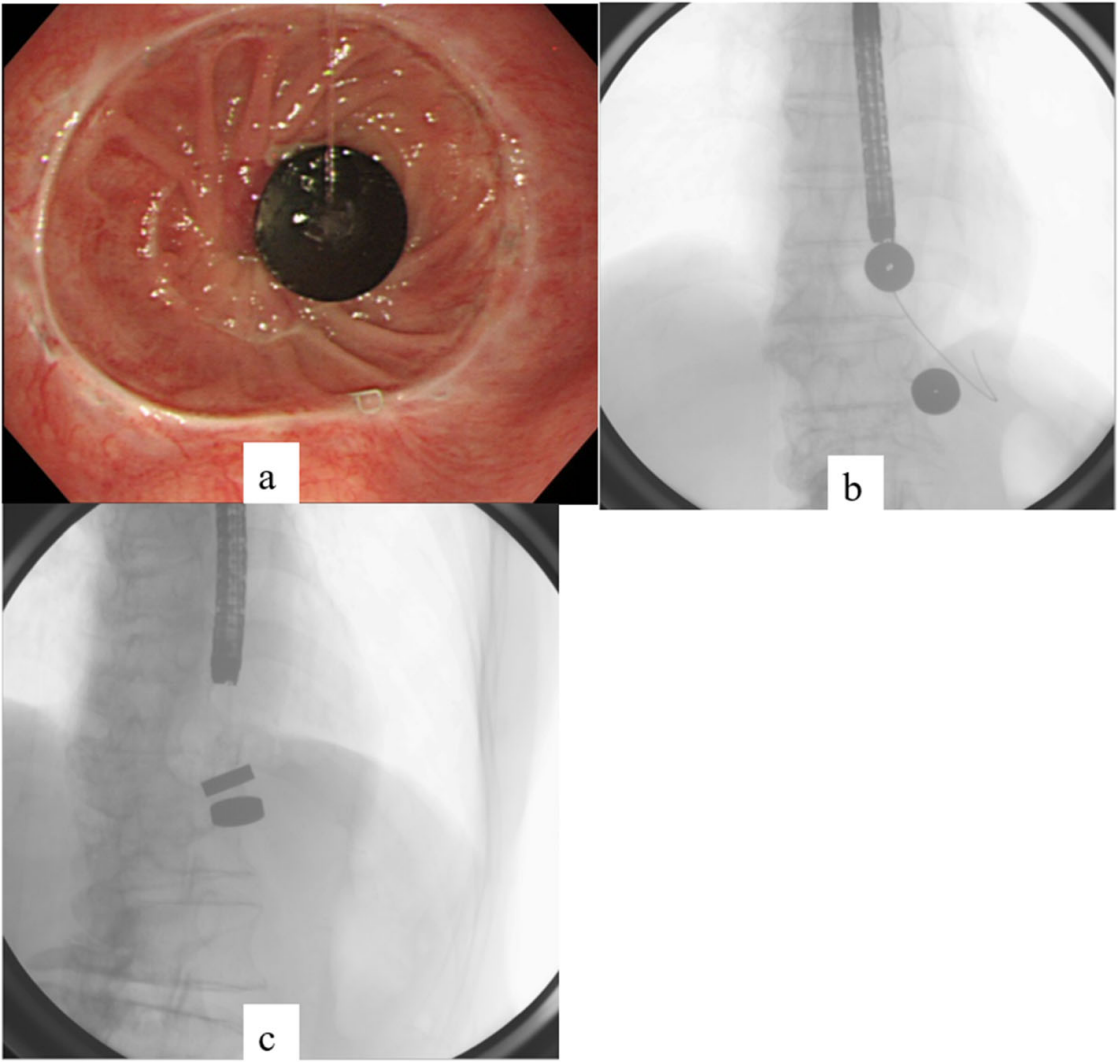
Magnetic compression anastomosis (placing the daughter magnet on the oral side of the stenosis). a Esophagogastroduodenoscopy. b Radiograph. c Radiograph showing adsorption of the two magnets in the side-to-side direction
